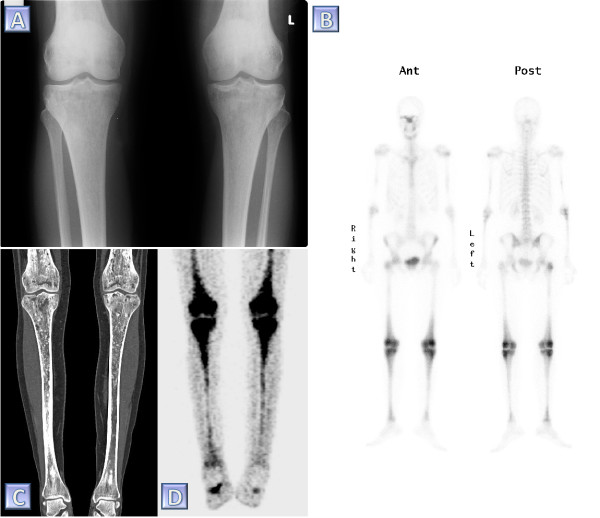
Diagnostic imaging in ECD. Various modalities in the skeletal assessment of a single ECD patient. (A) A plain radiograph of the knees demonstrating bilateral sclerotic changes in the femoral and tibial bones. (B) ^99m^Tc bone scintigraph taken prior to the diagnosis of ECD. Note the abnormally increased tracer uptake especially involving the periarticular region of the femurs and the tibiae. (C) Coronal reconstruction of a computed tomography study of the femurs and tibiae. Note the diffuse, irregular intra-medullary lytic-sclerotic pattern as well as the marked cortical thickening of the tibiae. (D) Coronal reconstruction of a positron emission tomography taken for the purpose of follow up 4.5 years pursuant the diagnosis of ECD. This study shows bilateral symmetric abnormally increased intra-medullary uptake of fluorodeoxyglucose in the femurs and tibiae.
